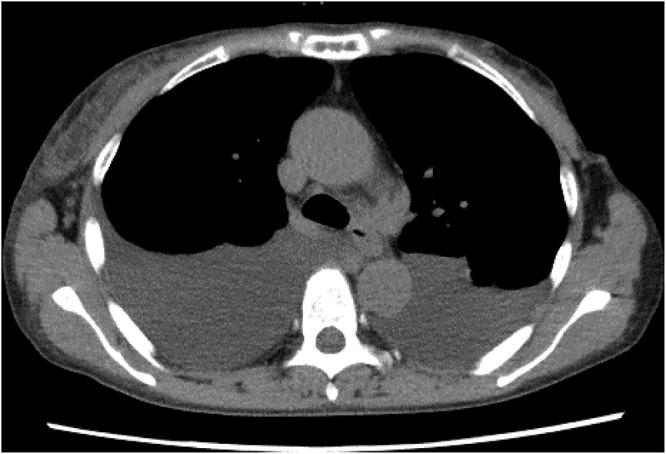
Chest computed tomography scan demonstrating large-volume bilateral pleural effusions. The effusions caused compression of the underlying lung parenchyma, contributing to respiratory compromise.
